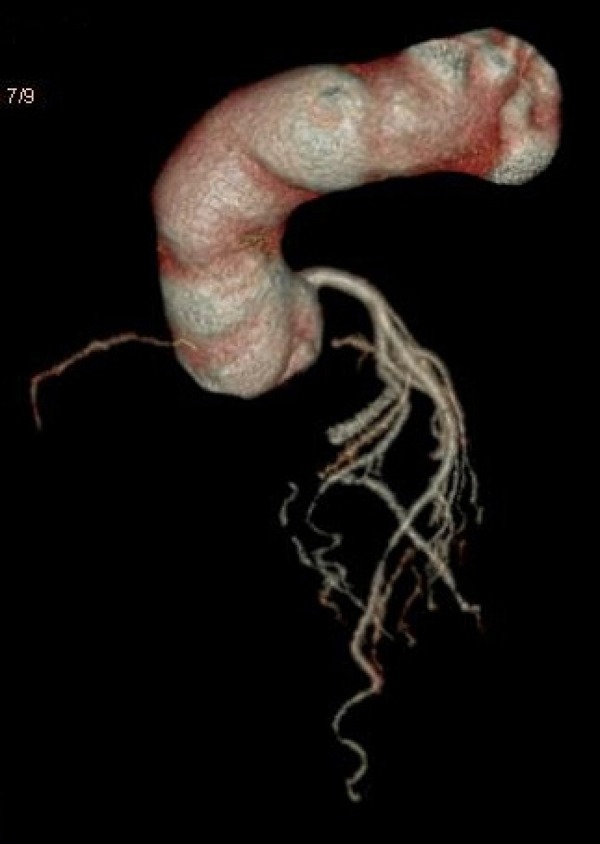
A follow-up CTA demonstrating that the right coronary artery was
